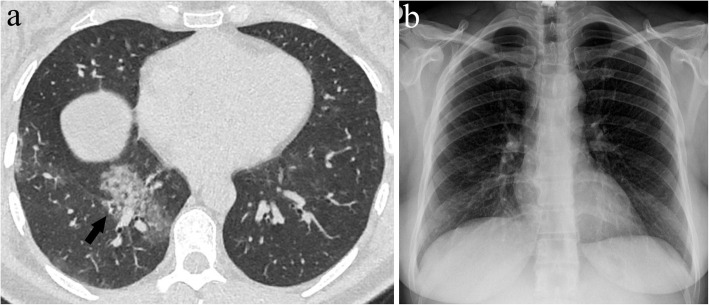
a, b A 43-year-old female presenting with fever and cough. Axial CT image at the level of lower lung zones (a) shows an area of consolidation in the right lower lobe below the level of the dome of the diaphragm (arrow). There is no observable opacity on the CXR image (b), revealing the limitation of CXR in demonstrating pathologies located in such blind spots
